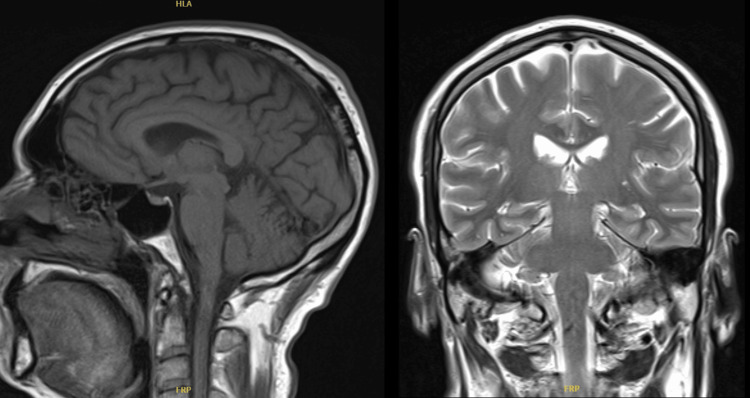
Brain MRI showing normal anatomy on both sagittal T1 (left) and coronal T2 (right) views. The ventricles are of normal size and shape, with no evidence of mass, hemorrhage, or structural abnormalities. The brain parenchyma, brainstem, and cerebellum appear intact and symmetrical, with no signs of oedema or lesions. Overall, the imaging is within normal limits. MRI: magnetic resonance imaging
